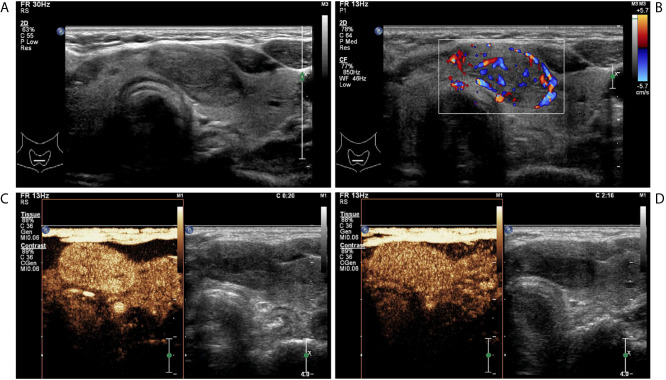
A follicular adenoma in a 63-year-old woman. (A) Greyscale ultrasound showed that there was a solid hypoechoic nodule in the isthmus of the thyroid, with regular margin, wider-than-tall shape, without calcification. The nodule was ACR TI-RADS category 4. (B) Color Doppler showed intranodular and peripheral vascularity. (C) Contrast-enhanced ultrasound revealed hyper-enhancement at peak (the 20th second after the injection of contrast agent), with regular hyper-enhanced ring and well-defined enhanced border. (D) Contrast-enhanced ultrasound revealed hyper-enhancement at the 136th second after the injection of contrast agent, which indicates later wash-out than the surrounding thyroid parenchyma.
